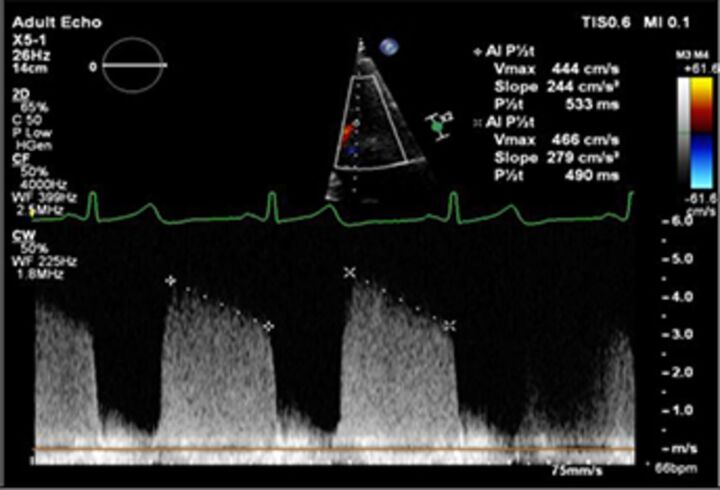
Easy If long, specific for mild	Affected by any factor altering aortic-LV pressure gradient Depends on alignment of Doppler with jet direction	>500 ms	<200 ms
